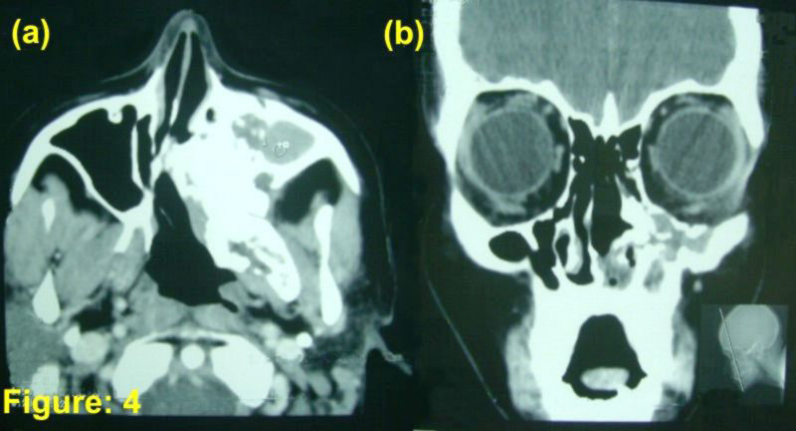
Axial and coronal contrast-enhanced paranasal sinuses and orbit computed tomography scan after completion of treatment (a, b). The scan is showing post radiation changes most likely to be fibrosis.
